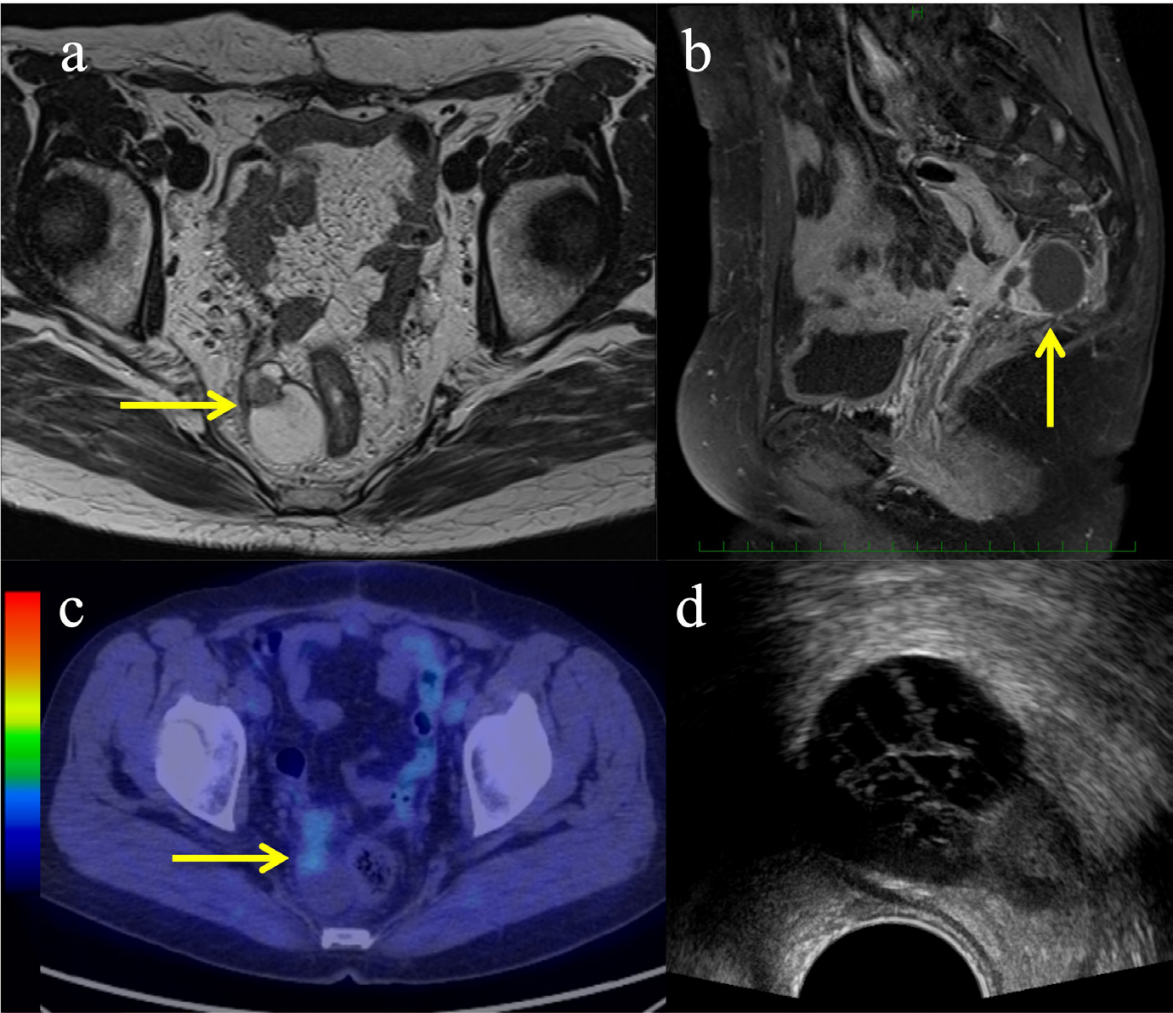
Pre-treatment images of the recurrent endometrial cancer. (a–b) Magnetic resonance imaging showing a tumour with a parenchymal and cystic component (yellow arrows): (a) Axial view of a *T*_2_-weighted image; (b) Sagittal view of a *T*_1_-weighted contrast-enhanced image; (c) Fluorodeoxyglucose uptake showing the parenchymal component of the tumour on positron emission tomography (yellow arrow); (d) Axial image of the transrectal ultrasound.
